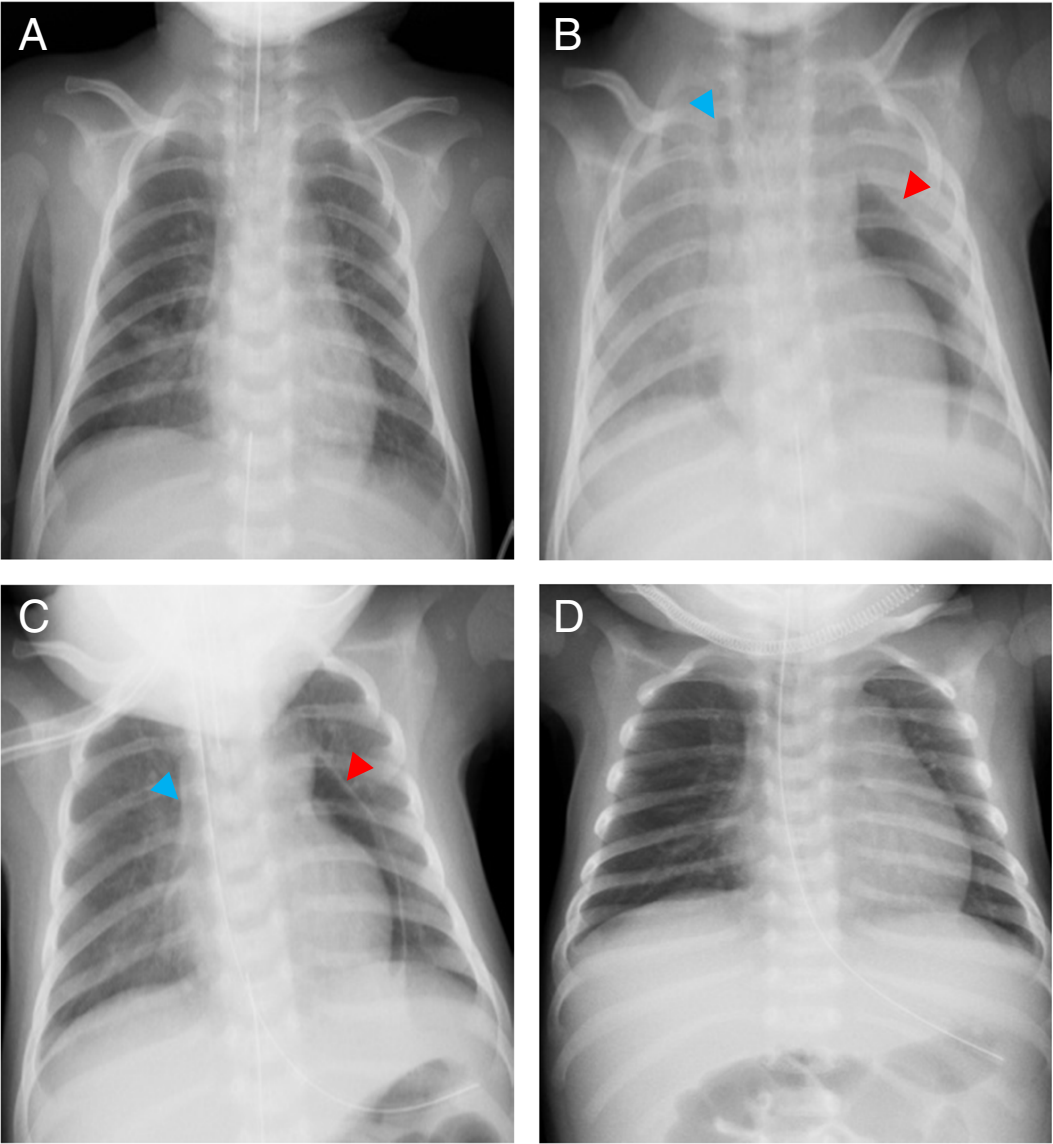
Chest X-rays in the operating room and in the intensive care unit. a Right after the pericardiocentesis, b before re-intubation approximately 2.5 h after admission to the ICU, c 10 min after re-intubation, and d after the drainage tube was removed. The red and blue arrows indicate pneumopericardium and pneumomediastinum, respectively
